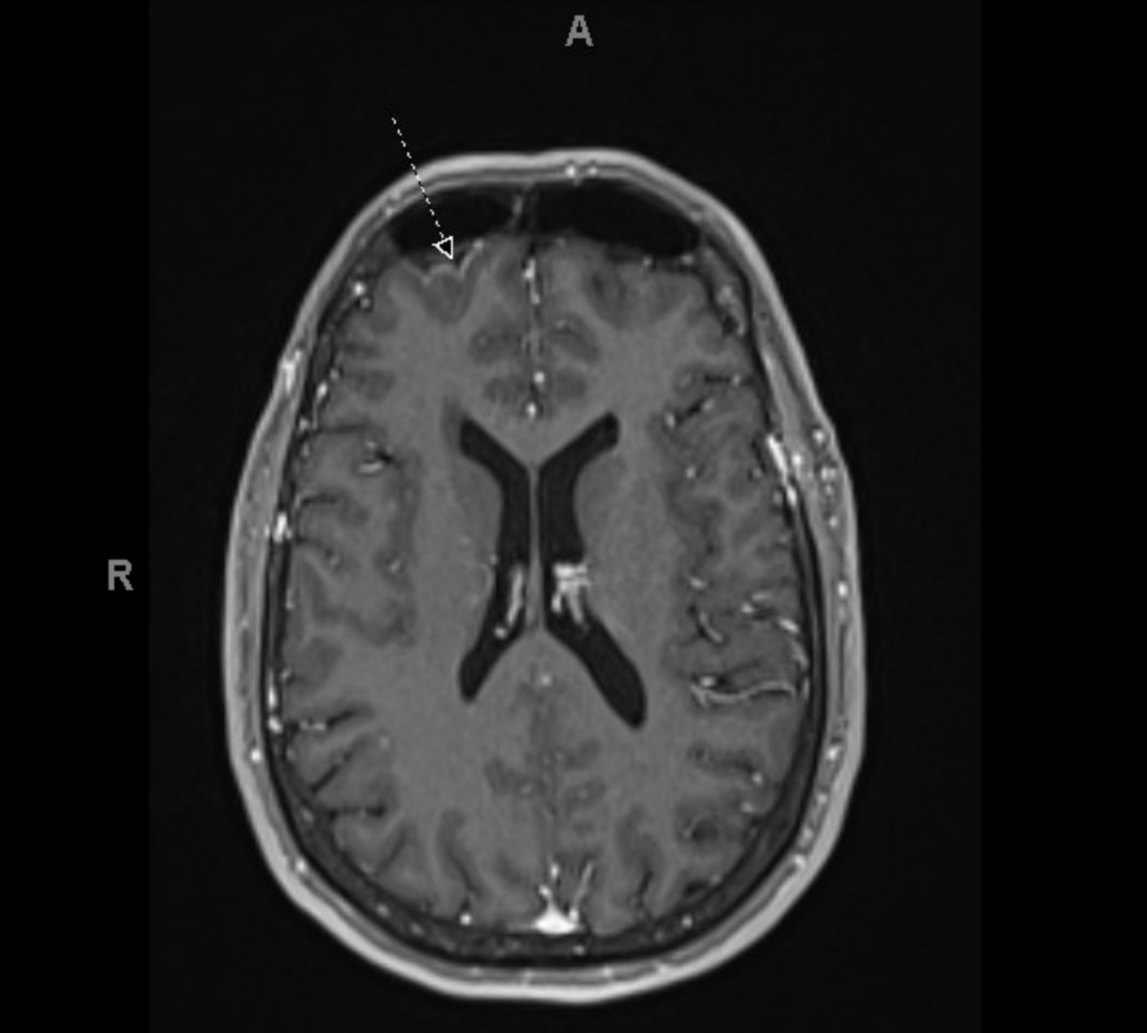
Brain MRI, FLAIR image, axial view: right frontal lobe pial enhancement (arrow) FLAIR - fluid-attenuated inversion recovery
